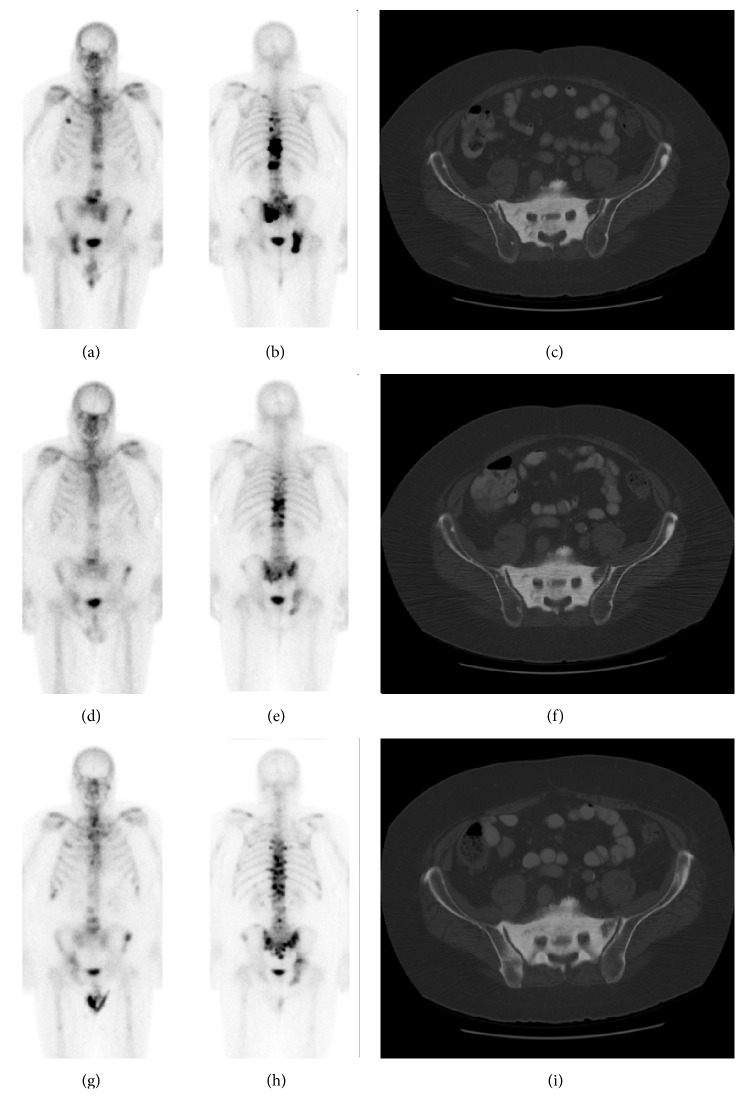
Case #1 of a 71-year-old man. Anterior and posterior bone scan, and CT at baseline (a, b, c), within 3 months after completion of Radium-223 (d, e, f), and 6–12 months after Radium-223 (g, h, i). Imaging findings are summarized in Table 1.
